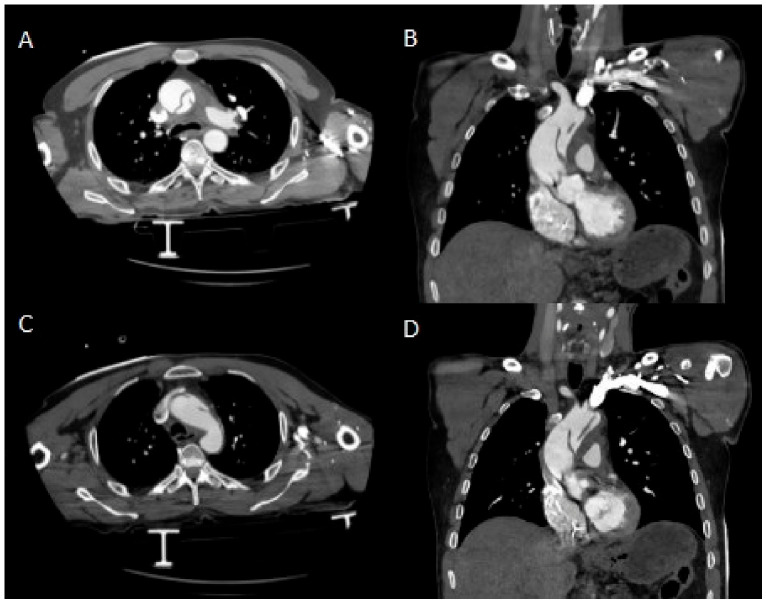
CT angiography of the thoracic aorta demonstrating acute aortic dissection. (A) Axial image at the aortic valvular plane shows the intimal flap originating at the level of the aortic root. (B) Coronal reconstruction confirms the extension of the dissection flap along the ascending aorta. (C) Axial image at the level of the aortic arch demonstrates the persistent intimal flap within the arch lumen. (D) Coronal reconstruction shows the dissection extending up to the origin of the left subclavian artery, with clear visualization of the true and false lumens.
